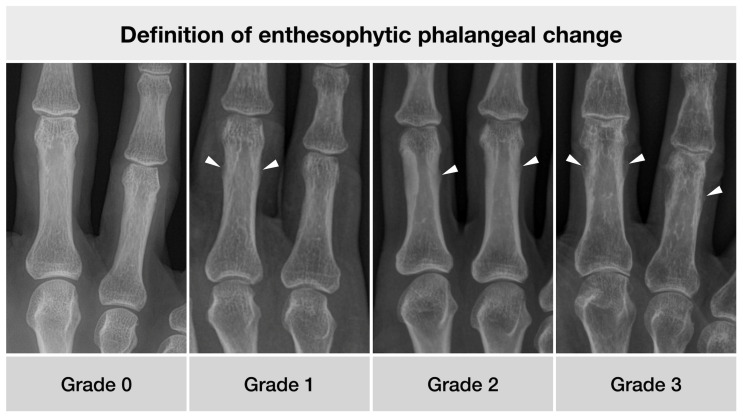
Anterior-posterior X-ray examples of the different grades of enthesophytic growth. Patients’ characteristics from left to right: 53 y/o female, 59 y/o male, 57 y/o female, 82 y/o male.
